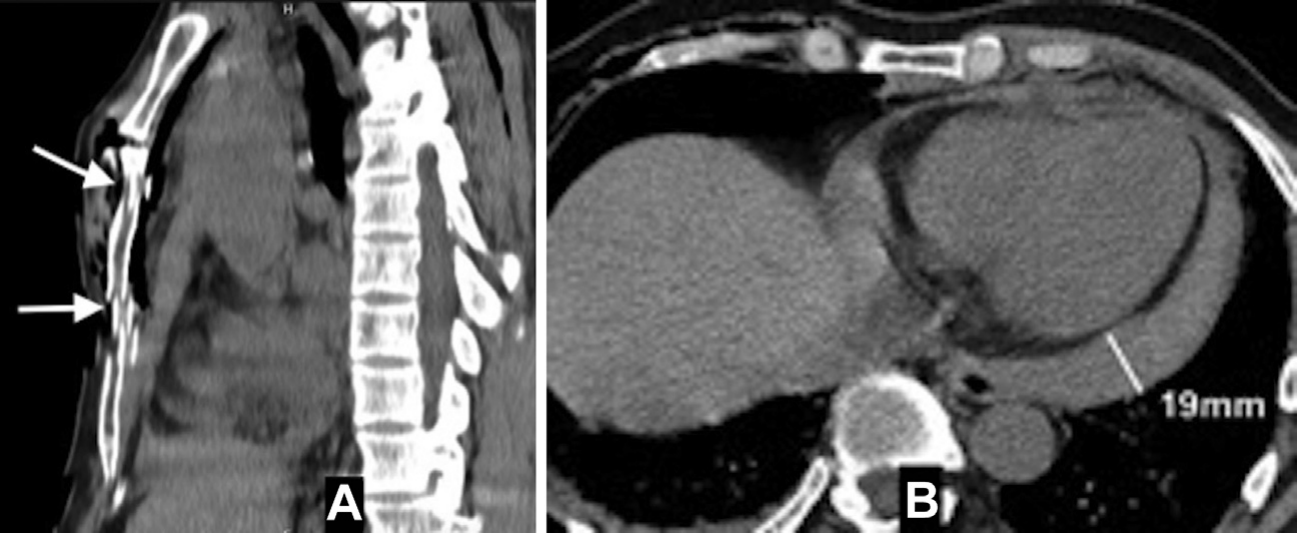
Computed Tomography Findings (A) Computed tomographic view demonstrating sternal fracture (arrowheads) and (B) 19-mm hemopericardium.
